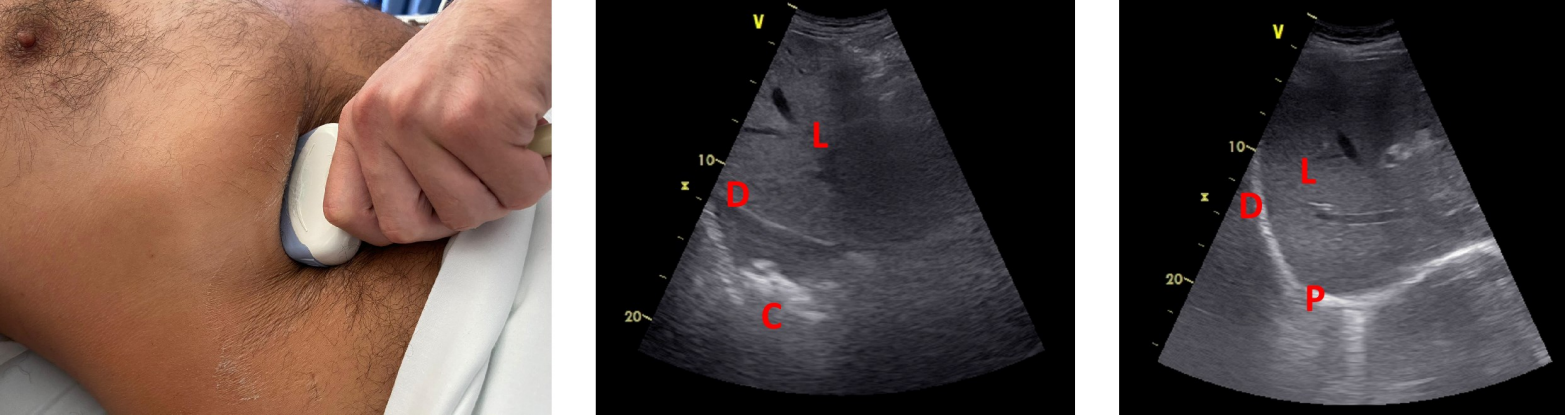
Positioning of the ultrasound probe in order to perform a trans-hepatic lung ultrasound (left panel); lung ultrasound findings in the same patient, showing the liver (L), diaphragm (D) and aconsolidated lower lobe of the right lung (C), as shown by the shred sign (middle panel); lung ultrasound findings in another patient without pneumonia, with visible pleura (P) and lung sliding (right panel).
